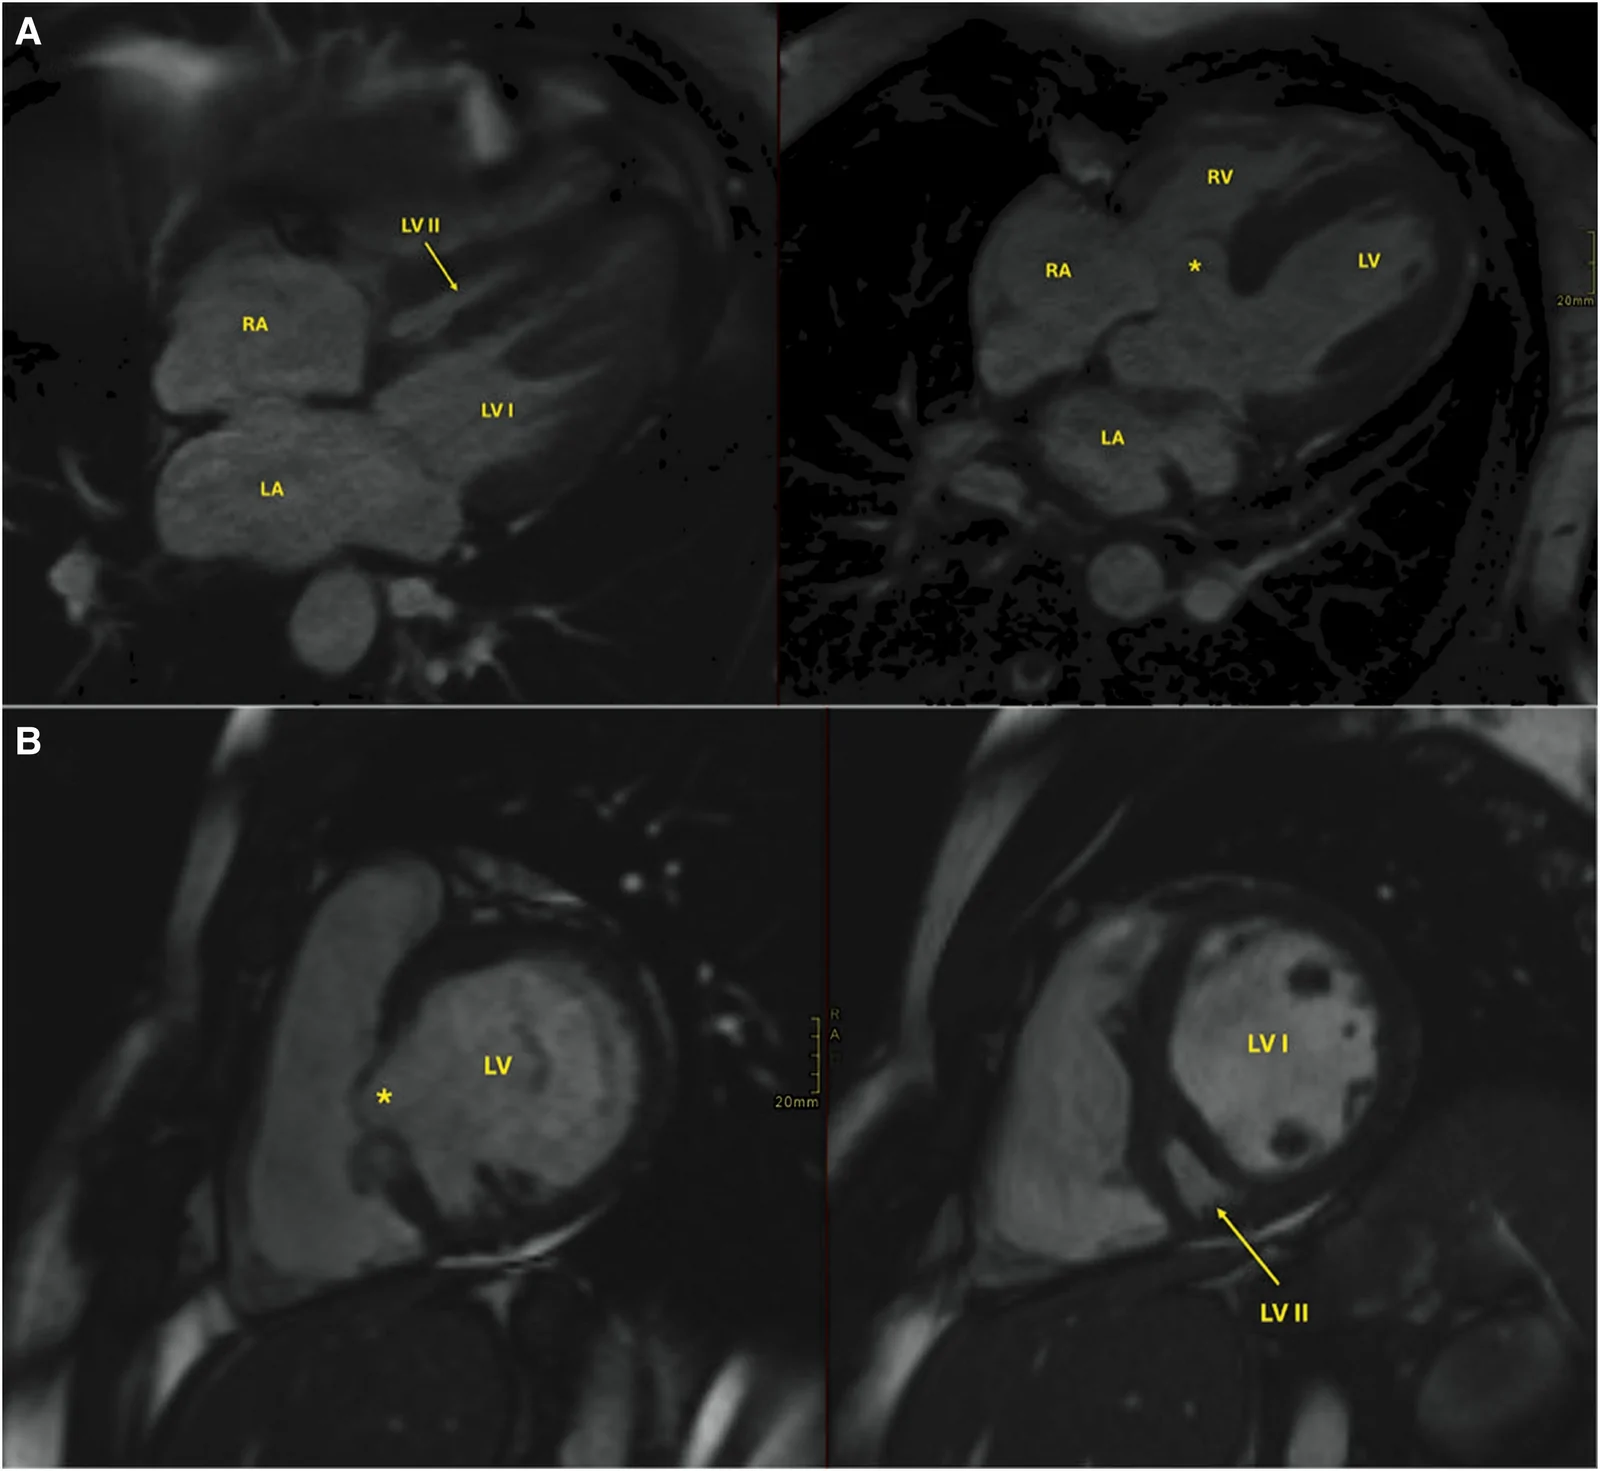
Cine cardiac magnetic resonance images demonstrating a perimembranous interventricular septal aneurysm (marked with asterisk) and a left ventricular accessory chamber (LV II). (*A*) Apical four-chamber view in systole. (*B*) Short-axis two-chamber view. LA, left atrium; LV/LV I, left ventricle/primary chamber of the left ventricle; LV II, accessory chamber of the left ventricle; RA, right atrium; RV, right ventricle.
